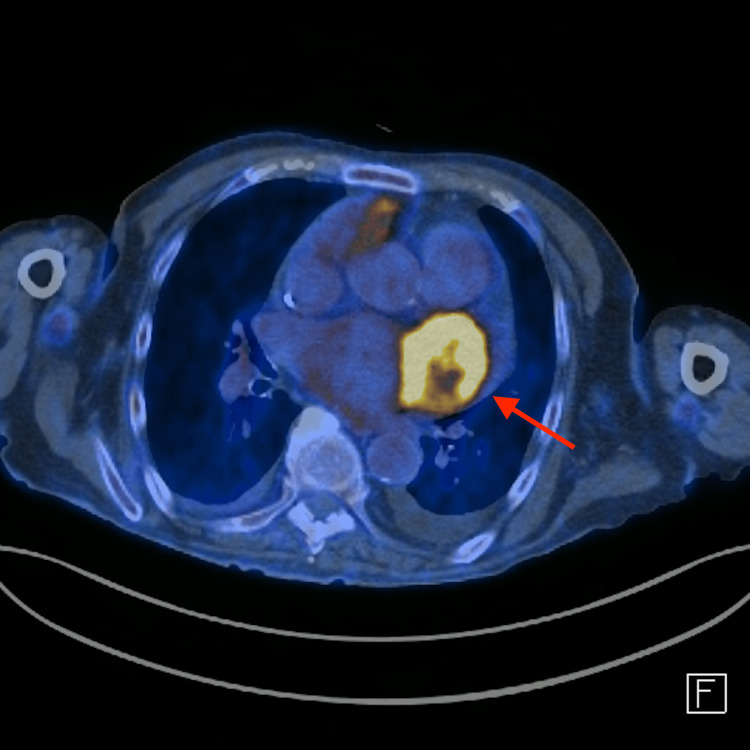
PET-FDG scan revealed a voluminous hypermetabolic mass in the heart, seemingly originating from the lateral aspect of the left auricle The mass measured approximately 56 × 48 mm in its largest axial axes, with a maximum standardized uptake value (SUVmax) of 14.3. The mass exhibited anatomical and functional characteristics consistent with a malignant neoplastic mass with a high metabolic rate.
